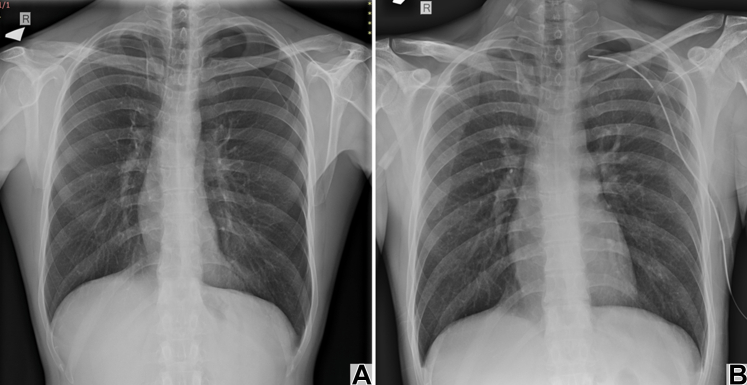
A, B, Chest radiographs from initial presentation 3 y earlier: (A) large, left-sided pneumothorax with partial lung collapse during the patient’s hospitalization, and (B) chest tube in place, with incomplete reexpansion of the left lung.
